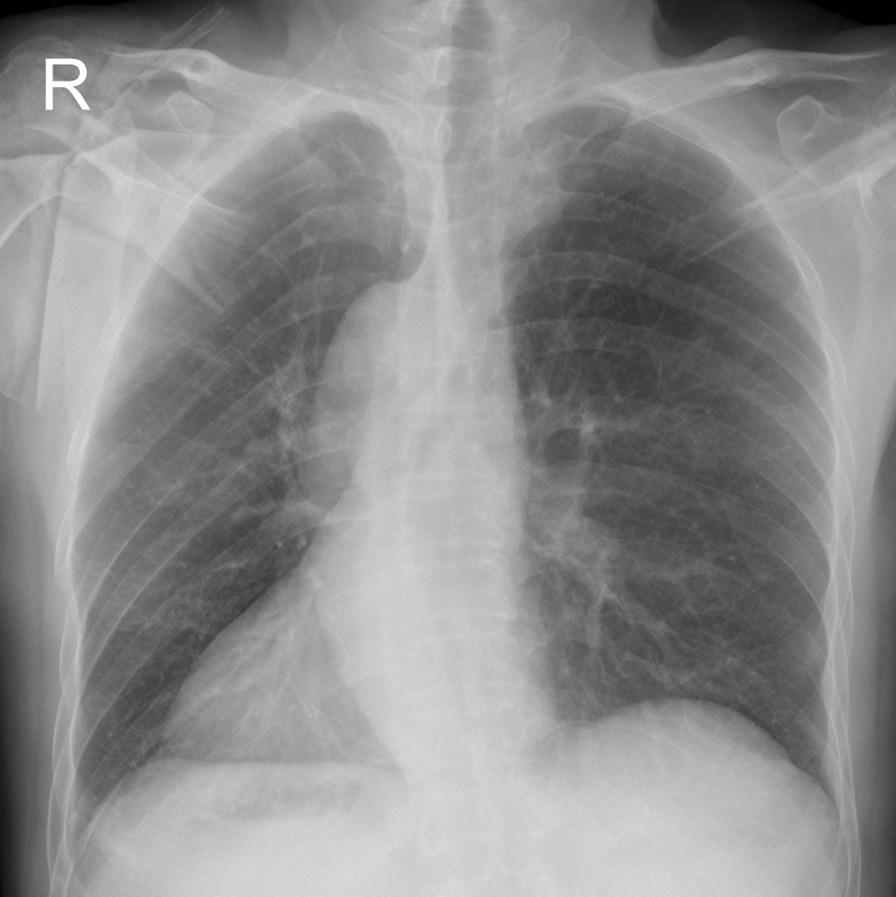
Chest radiograph on admission shows dextrocardia and positioning of the aortic arch on the right side, no tumor shadow could be seen
